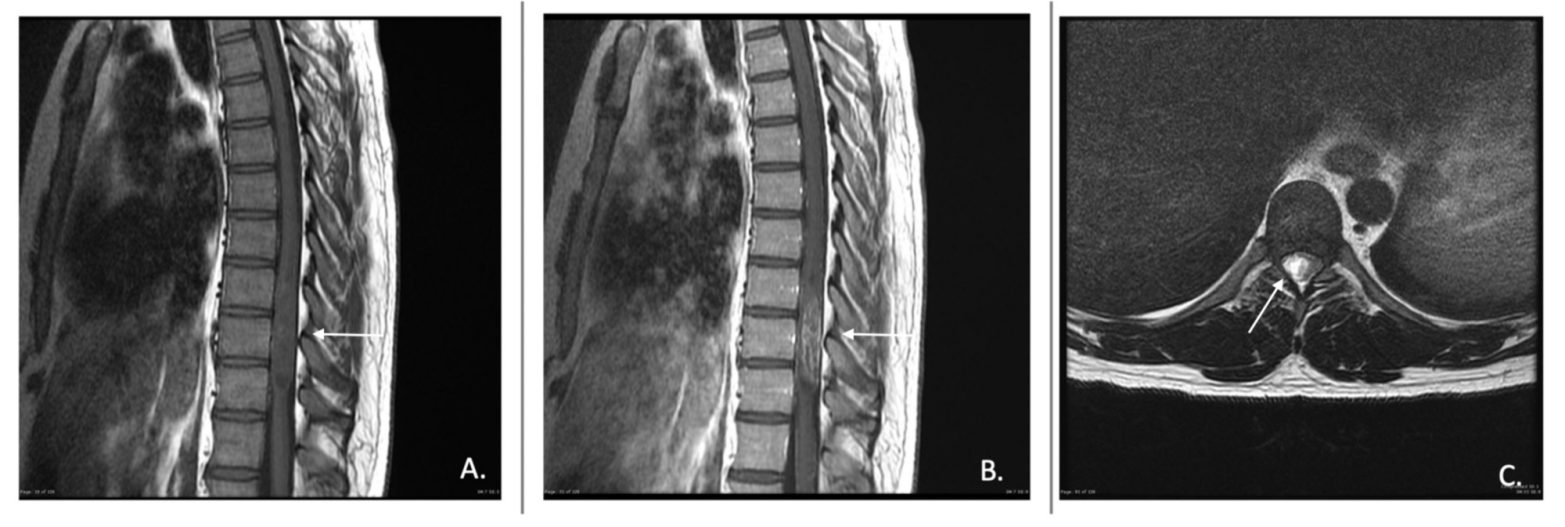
Pilocytic astrocytoma of the thoracic spine. A) T1-weighted sagittal pre-contrast image, B) T1-weighted sagittal post-contrast image, and C) T2-weighted axial slice showing displacement of thoracic cord by area of hyperintensity consistent with spinal astrocytoma. Photo Reference: Courtesy of Assoc. Prof. Frank Gaillard, Radiopaedia.org, rID: 19536
